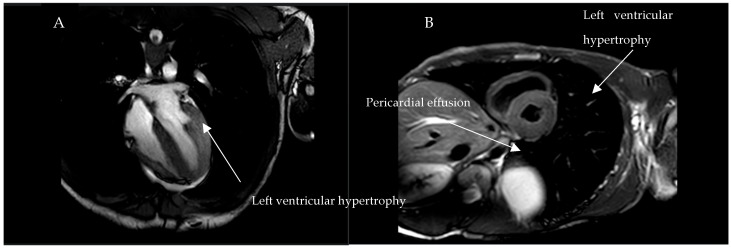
Cardiac MRI of male patient. (A) Concentric thickening of the walls of the left ventricle; (B) Circumferential pericardial effusion flap.
